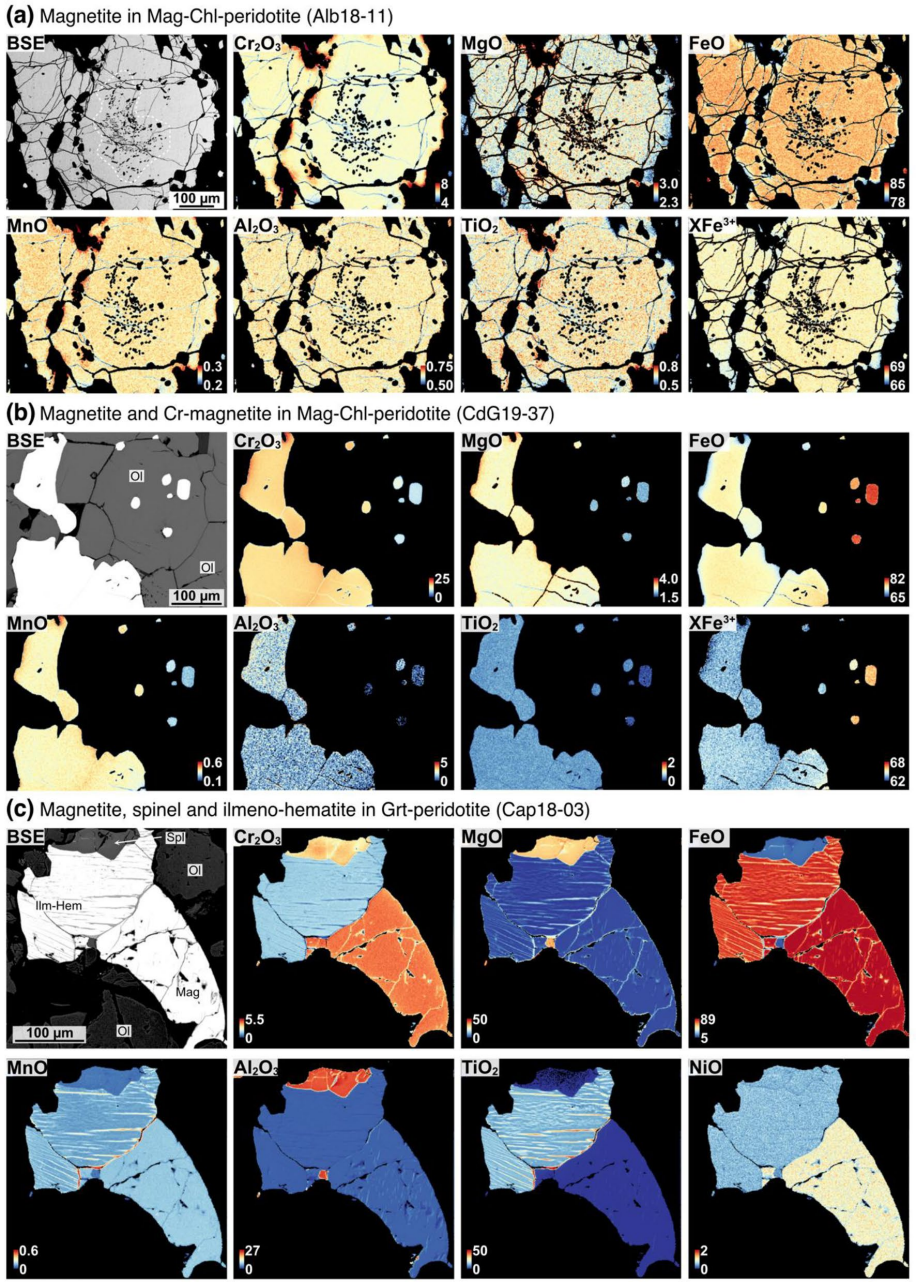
Compositional X-Ray EPMA maps of oxide grains, showing concentrations in oxide wt.%. a Subhedral magnetite in Mag-Chl-peridotite of Alpe Albion, showing uniform composition for inclusion-rich core and inclusion-free rim. b Inclusion and subhedral Cr-magnetite in Mag-Chl-peridotite of Cima di Gagnone. c Intergrowths of magnetite, ilmeno-hematite, and retrograde spinel in former Grt-peridotite of Alpe Capoli
